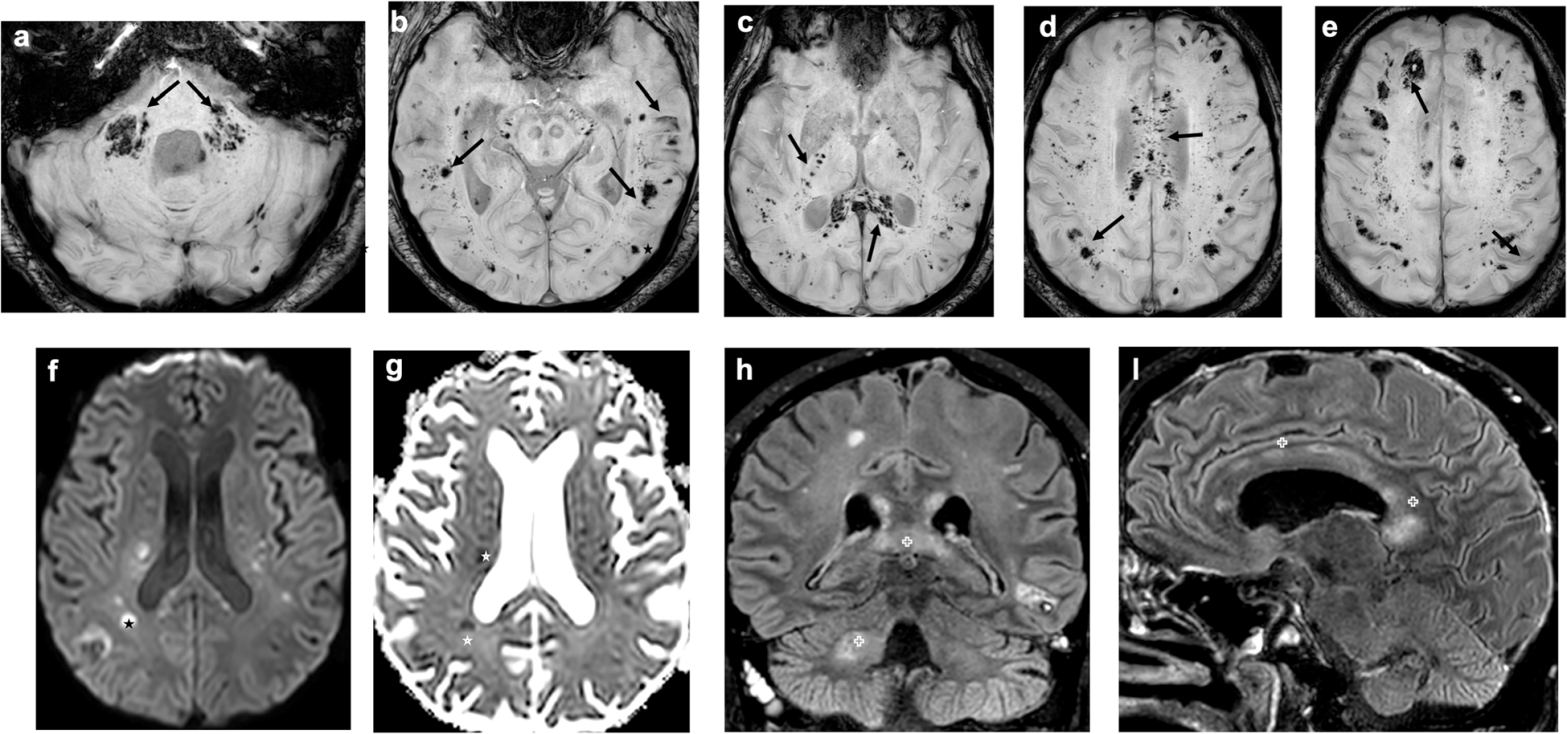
Axial SWI (a–e), axial diffusion (f), apparent diffusion coefficient (ADC) (g), coronal (h), and sagittal (i) FLAIR-weighted MR images: multiple infra and supratentorial white matter microhemorrhages (arrows), associated with FLAIR (cross) and diffusion (star) hyperintensities
